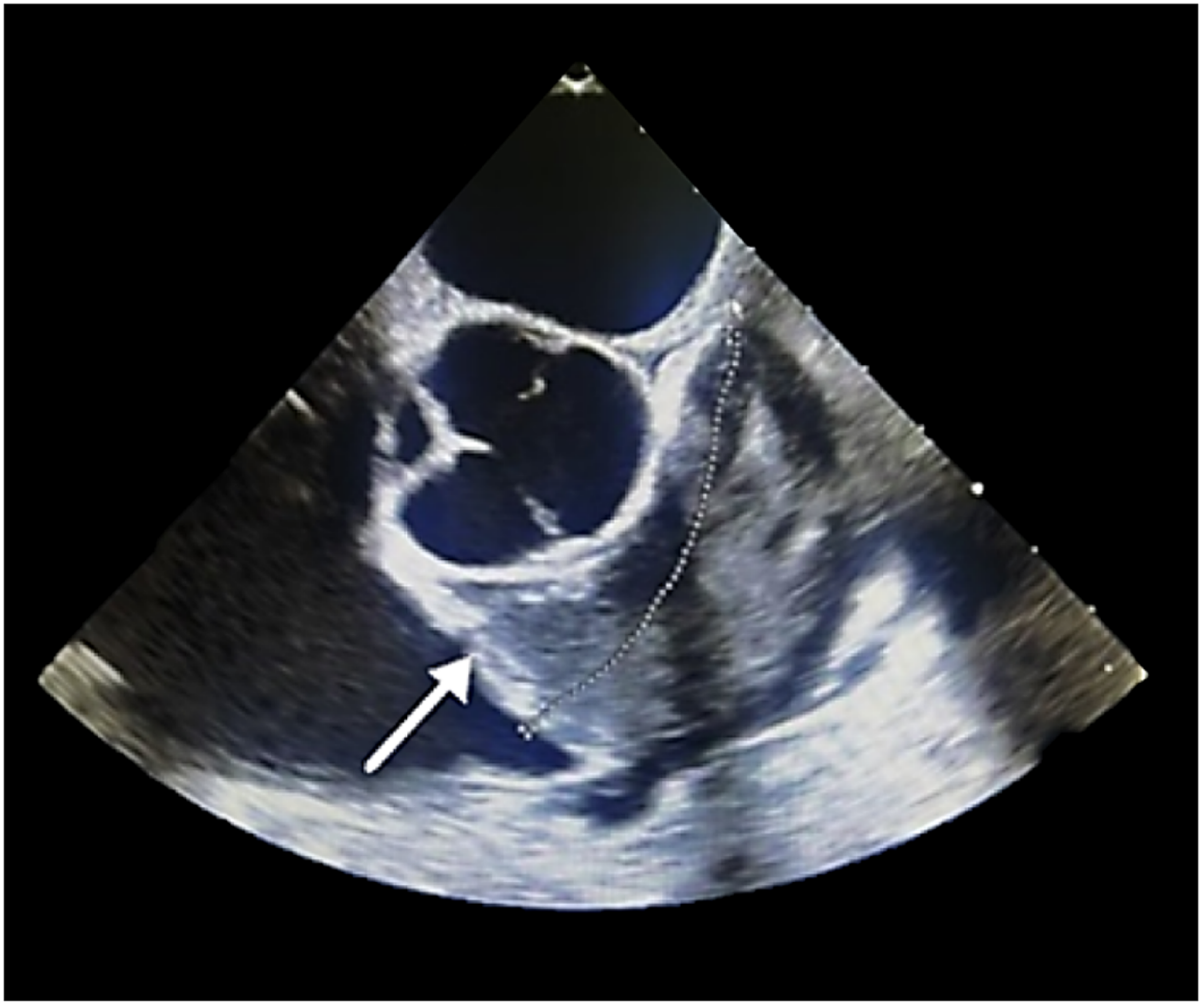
Transesophageal Echocardiogram (short axis view) of a 44‐year‐old patient diagnosed with ISPA. This transesophageal echocardiogram reveals a large, multilobular, broad‐based mass (indicated by the arrow) measuring approximately 5.7 × 2.7 cm. The mass demonstrates tissue‐like echotexture with vascularity, originating from the arterial aspect of the pulmonary valve (PV) leaflet. It extends into both the main pulmonary artery (MPA) and right pulmonary artery (RPA), causing significant systolic turbulence in these regions. This flow disturbance contributes to moderate pulmonary stenosis (PS) with a peak gradient of 59 mmHg. Additionally, the left ventricular ejection fraction (LVEF) is moderately preserved at 55%, while the right ventricular ejection fraction (RVEF) is significantly reduced at 30%, indicative of compromised right ventricular function. ISPA, intimal sarcoma of the pulmonary artery; LVEF, left ventricular ejection fraction; MPA, main pulmonary artery; PS, pulmonic stenosis; PV, pulmonary valve; RPA, right pulmonary artery; RVEF, right ventricular ejection fraction.
